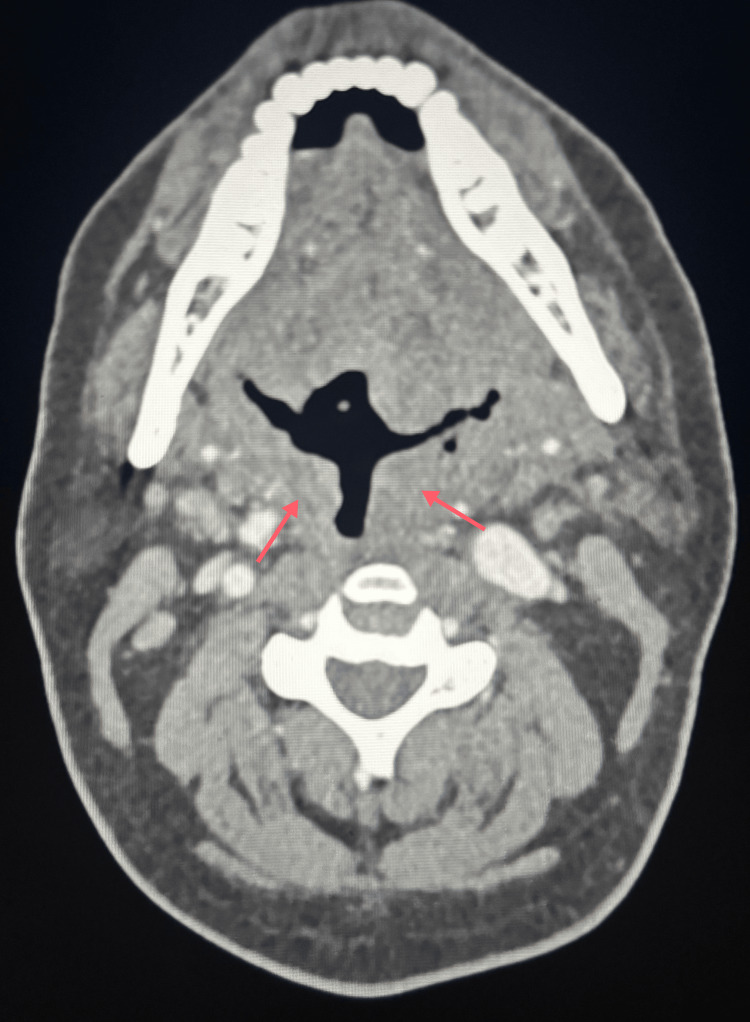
Axial contrast-enhanced CT of the neck demonstrating oropharyngeal soft tissue thickening Axial contrast-enhanced CT image of the neck demonstrating moderate soft tissue thickening along the left oropharynx (red arrows), with involvement of adjacent structures and mild narrowing of the oropharyngeal airway. No discrete source of hemorrhage or rim-enhancing collection is identified. CT: computed tomography
